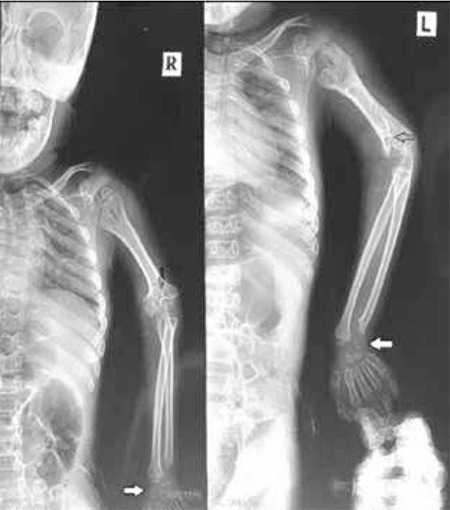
X-ray appearance of the upper extremities showing shortening of the humerus and calcifications at the elbow (black arrows). Ulnar hypoplasia and distal radio-ulnar dysplasia are present in both hands (white arrows). L: Left side, R: Right side of the patient
